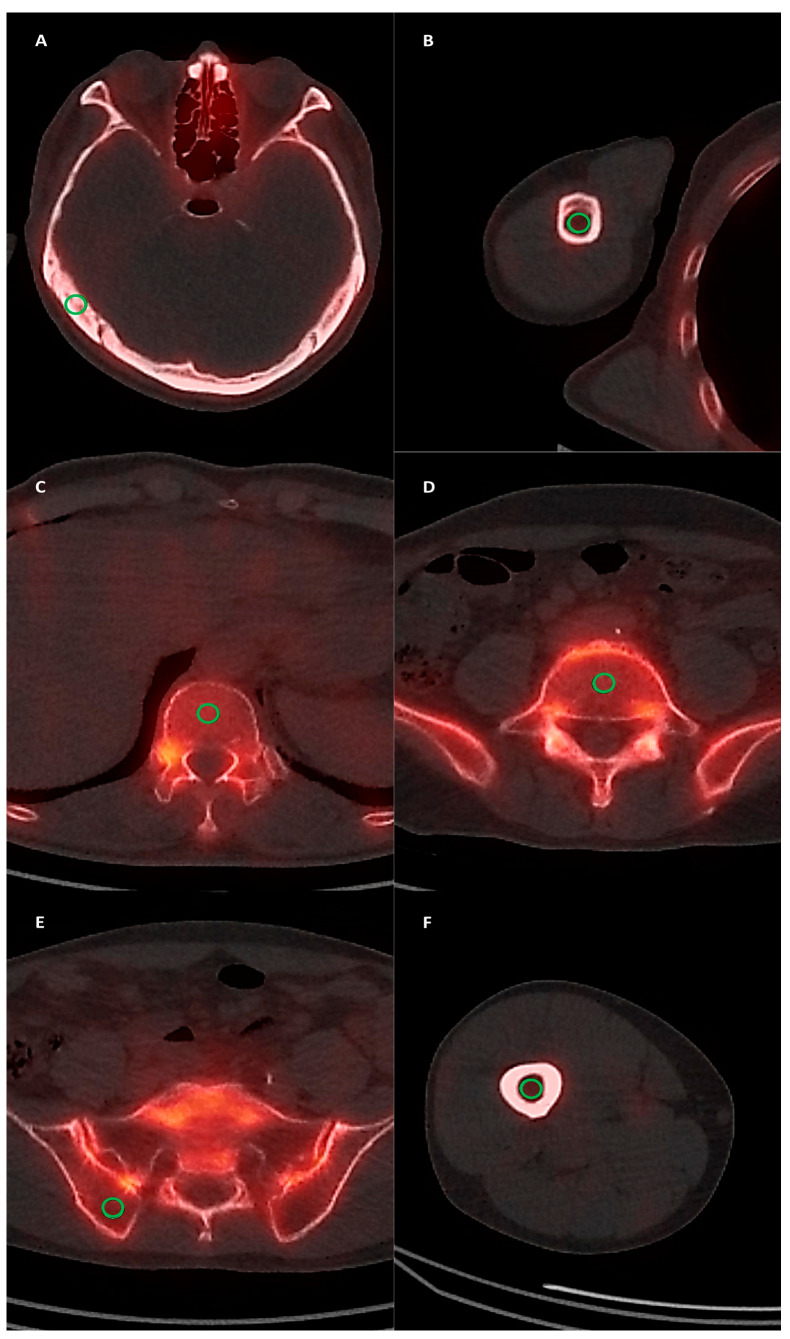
Examples of normal bone uptake measurements at the skull (A), humerus (B), thoracic spine (C), lumbar spine (D), iliac bone (E), and femur (F). A 1 cm-sized spherical VOI was manually drawn (green circle), and the SUVmax and SUVmean of the VOI were measured.
